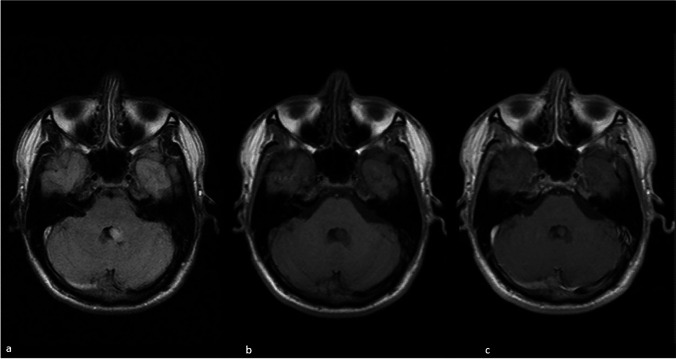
(a) Ubiquitous hyperintense signal of the lesion in the axial FLAIR MRI reveals a developing lesion in IV ventricle. (b) Axial T1 weighted MRI at the level of cerebellopontine peduncle reveals corresponding hypointense signal of the lesion. (c) Apparent ubiquitous T1 relaxation time shortening of the lesion in axial post-gadolinium sequences
